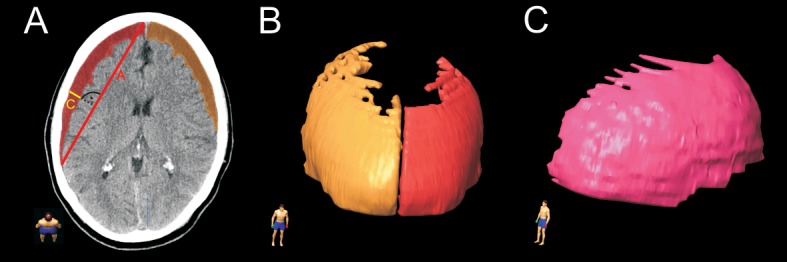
A. Preoperative axial CT-scan with both sided subdural hematomas (SDH). Computer-assisted volumetric measurement was performed by hand tracing each hematoma form in each slice as shown in red and orange colour. ABC/2 measurement technique was performed as following: A is the length connected each corner of the SDH, B is 90° to A and C is the height calculated by the slice thickness with visible hematoma. B. 3D frontal reconstruction of computer-assisted volumetric measurement of both sided SDH. C. 3D lateral reconstruction of computer-assisted volumetric measurement of single sided SDH.
